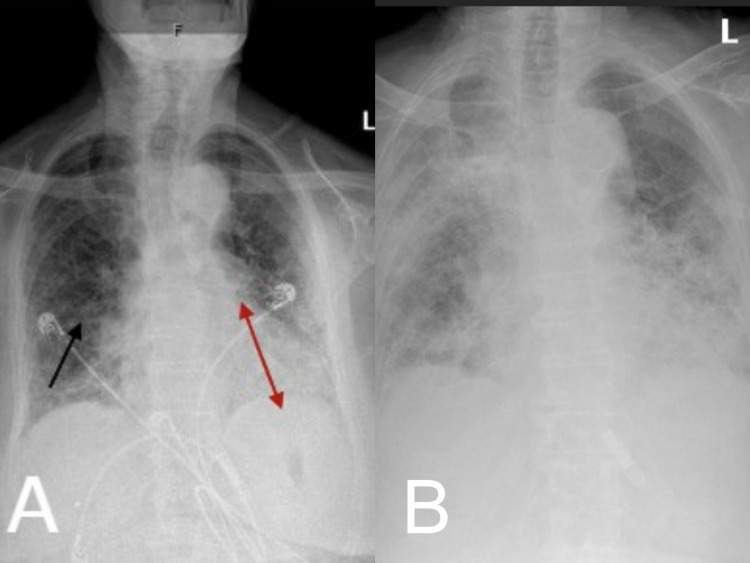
Chest x-ray: (A) showing lobar pneumonia (area under the double headed arrow); (B): showing diffuse pneumonia on hospital day 13.
